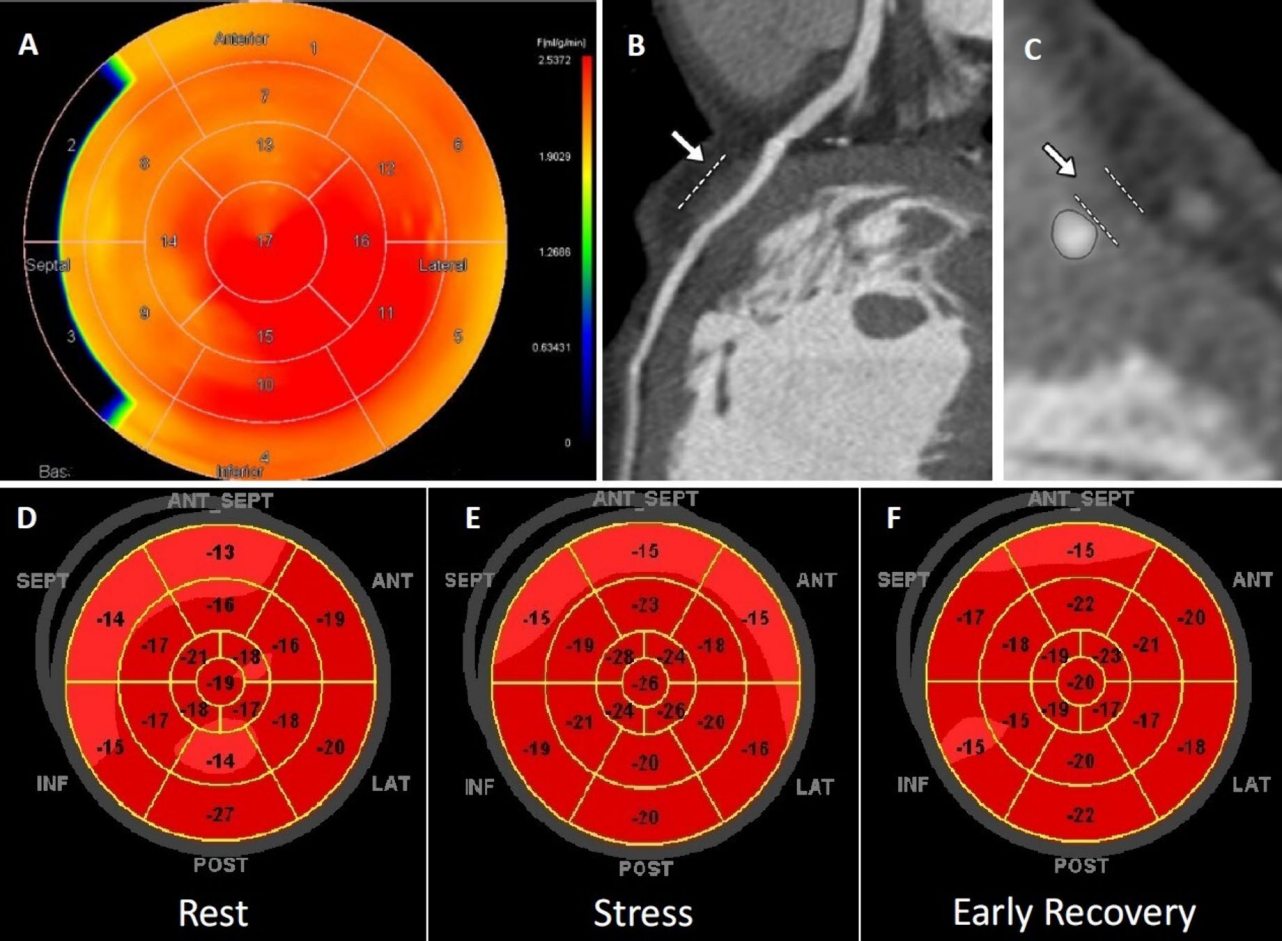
Positron emission tomography (PET), computed tomography angiography (CTA), and dobutamine stress echocardiography (DSE) of a patient with myocardial bridging (MB) in the middle left anterior descending (LAD) coronary artery. A polar map of stress myocardial perfusion measured using 15-Oxygen water PET shows normal stress myocardial blood flow (A). Multiplanar reconstruction (B) and cross-sectional (C) images of a MB in the mid-LAD artery. White arrows and lines demonstrate the myocardium overlying the artery. Representative polar maps of peak systolic longitudinal strain at the rest (D) peak dobutamine stress (E) and early recovery (F) phases. Myocardial strain is homogeneous throughout the test
